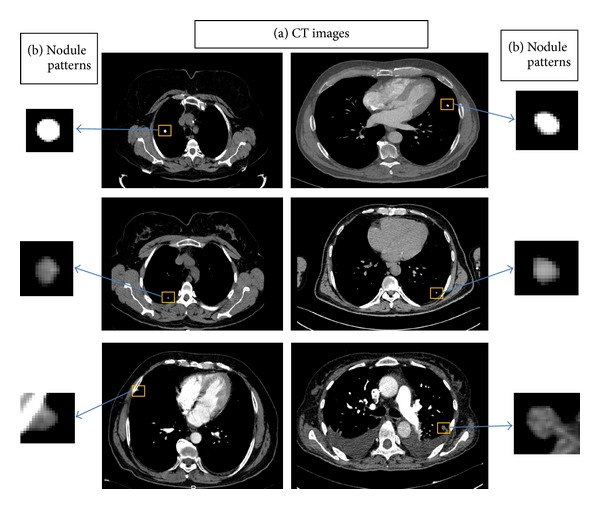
Two-dimensional image samples: (a) CT images and (b) pulmonary nodule patterns.
